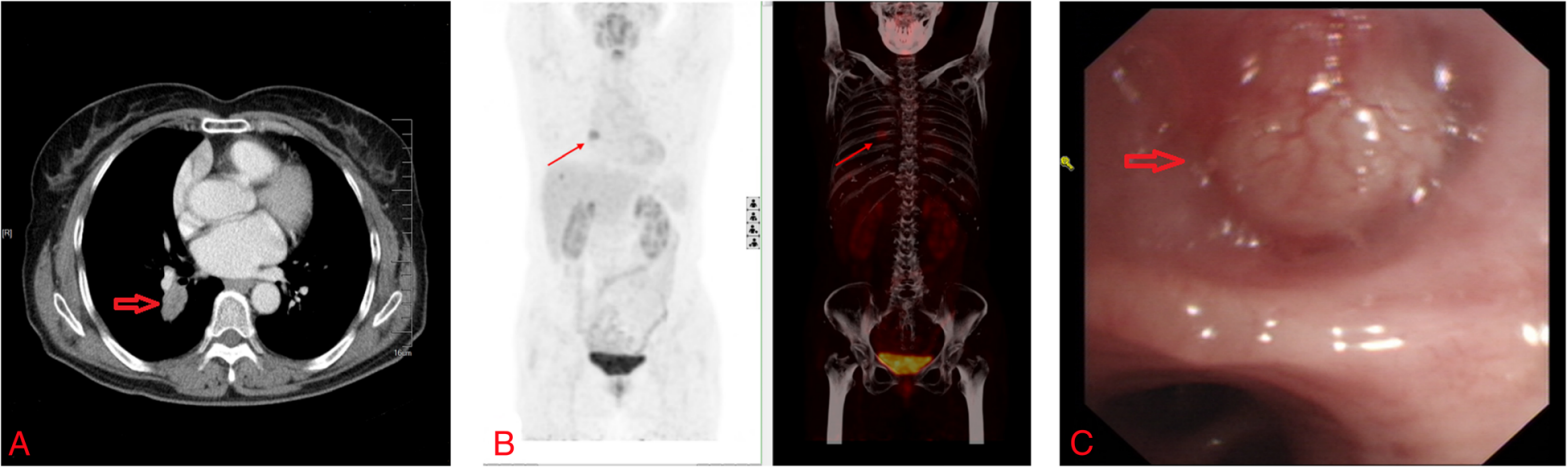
A Chest CT scan revealed an interval enlargement of a 2.8-cm dorsal segment of the right lower lobe mass (red arrow). B PET-CT showed central hypermetabolic activity only in the right lower lobe mass (red arrow). C Bronchoscopy revealed a dorsal segment of the right lower lobe with a possible cancerous mass (red arrow)
